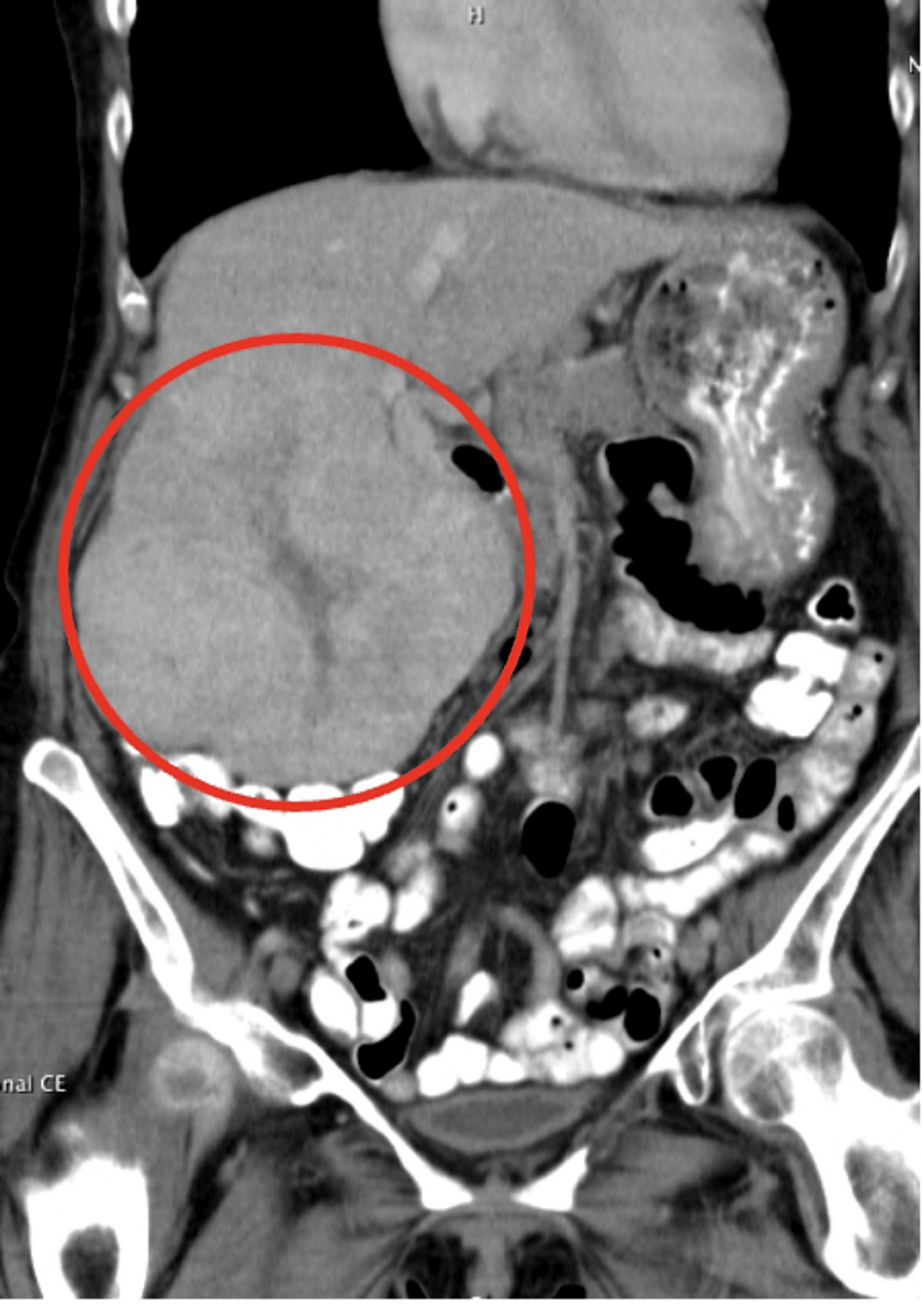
CT scan showing vascular mass in the central portion of the liver 15.5 x 12.0 x 13.5 cm vascular mass denoted with the arrow in the central portion of the liver and involving the right and left hepatic lobes extending inferiorly into the upper pelvis. The lesion demonstrates local mass-effect.
